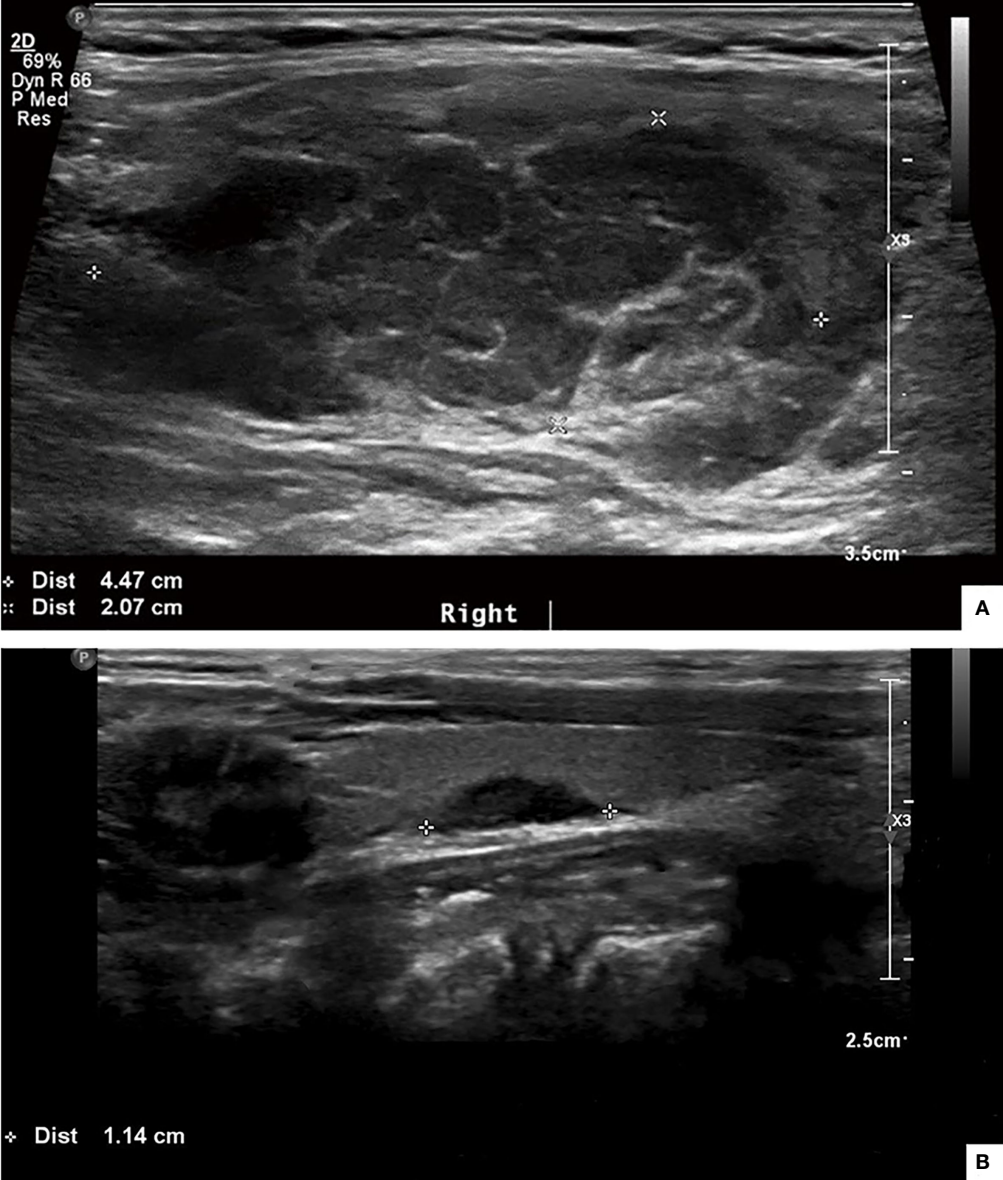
Two typical ultrasonographic types of thyroid LCH. The diffuse type: a large hypoechoic area covering 80%-90% of the right thyroid lobe (A). The nodular type: a hypoechoic nodule with irregular contour on the left thyroid lobe (B).
